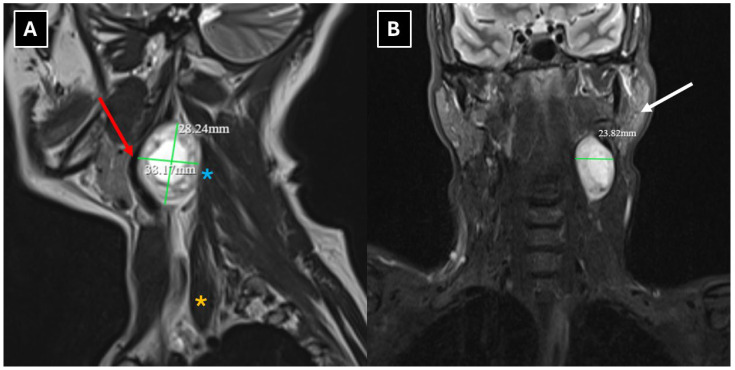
MRI scan of the neck. (A): Sagittal T2-weighted and (B): coronal STIR images show a well-defined mass located in the dorsal part of the carotid space at the C2-C4 level, with a ventral displacement of the internal and external carotid arteries. The mass measured 28 × 23 × 38 mm (AP × LL × CC). The mass demonstrates heterogeneous intermediate intense appearance, with peripheral and central areas of marked hyperintensity, suggestive of a peripheral solid component and central cystic degeneration. Red arrow—internal carotid artery; white arrows—parotid gland; orange asterisk—sternocleidomastoid muscle; blue asterisk—middle scalene muscle.
